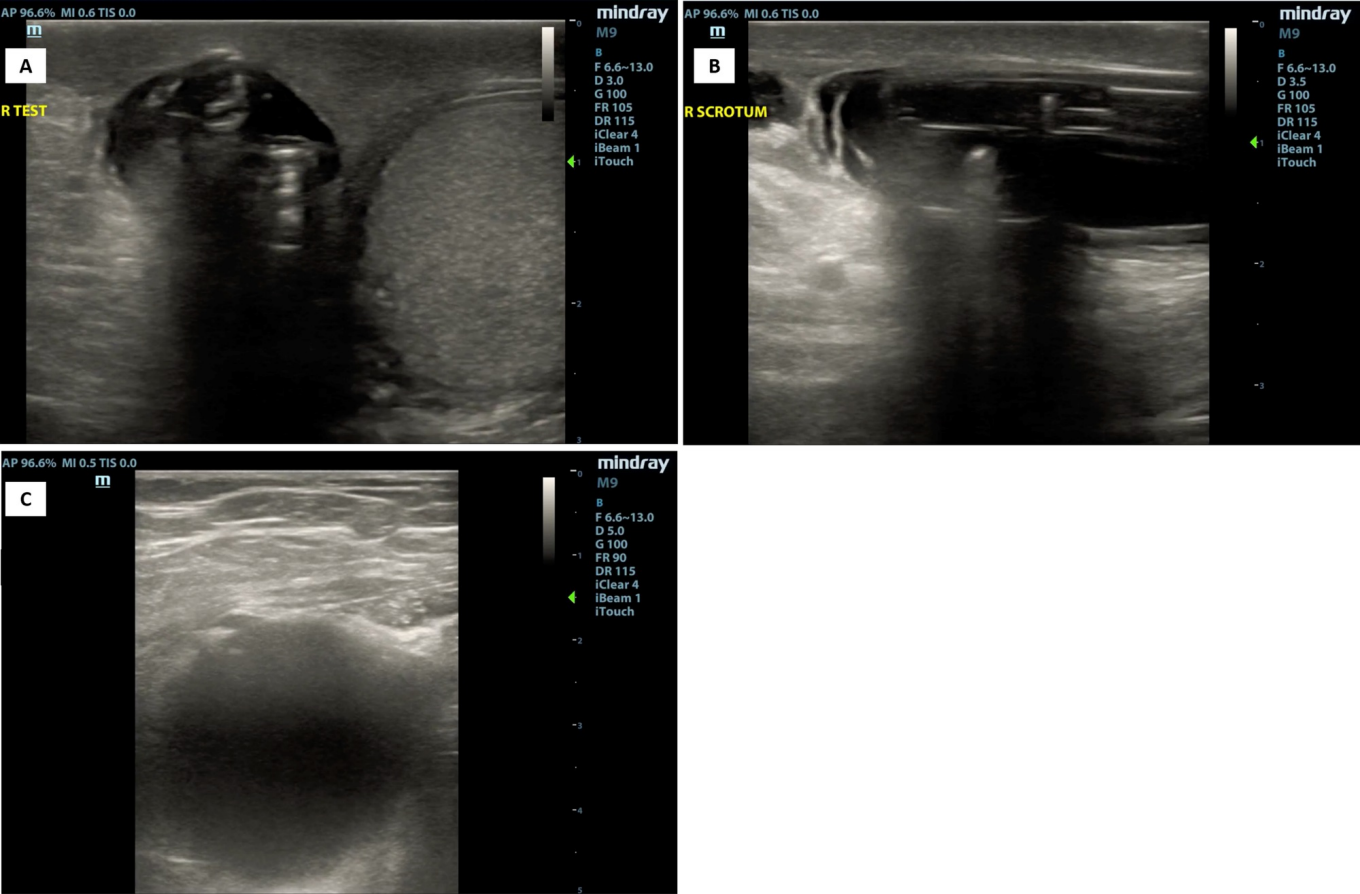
Ultrasound images of artificial urinary sphincter device Ultrasound of the right testicle demonstrates the artificial urinary sphincter location and placement seen in the long-axis view (A) and the short-axis view (B). Suprapubic view (C) also shows a visualization of the balloon reservoir.
